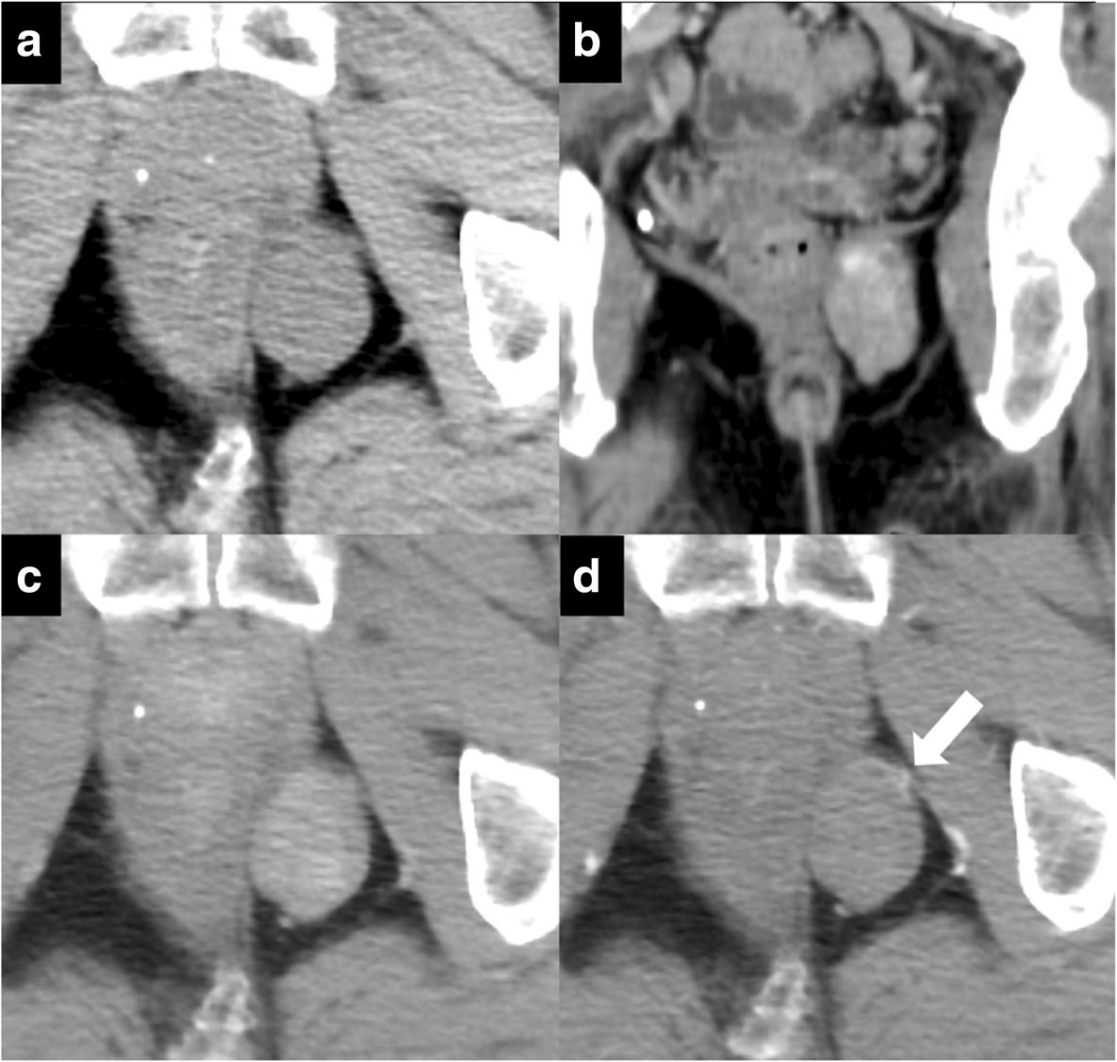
Axial and reconstruction of coronal contrast-enhanced CT. a A 5-cm, well-defined solid mass in the left ischiorectal fossa abutting the left anal wall and extending into the inferior perineum. b Coronal image: contrast-enhanced CT showing intense heterogeneous enhancement. c Axial image in the delayed phase. Delayed persistence of the mass is revealed. d Axial image in the early phase. A feeding vessel is seen near the mass (white arrow)
